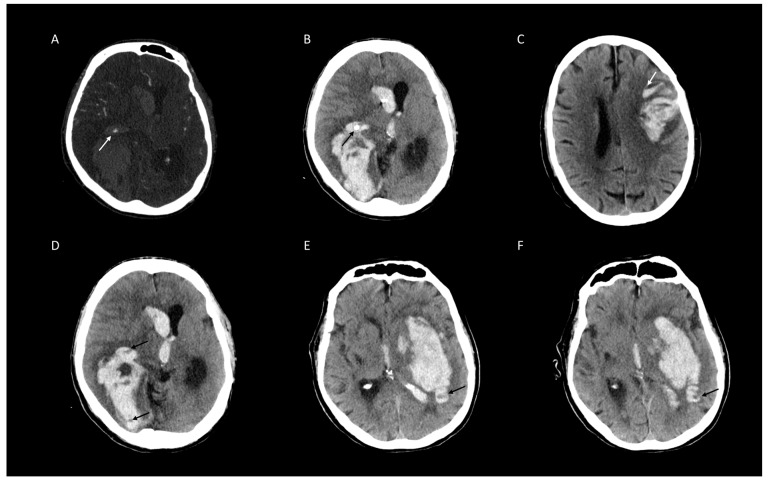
Representative examples of disagreed ratings of four non-contrast computed tomographic (NCCT) markers and Spot Sign (SS) on CT-angiography (CTA) for intracerebral hemorrhage expansion. (A) SS on CTA (white arrow) mistaken for intraventricular plexus calcification (black arrow) (B). (C) Blend sign (white arrows) mistaken for Fluid Sign1. (D) Swirl Sign mistaken for Hypodensities (black arrow). (E) Hypodensities (black arrow) mistaken for Swirl Sign (F) [6].
